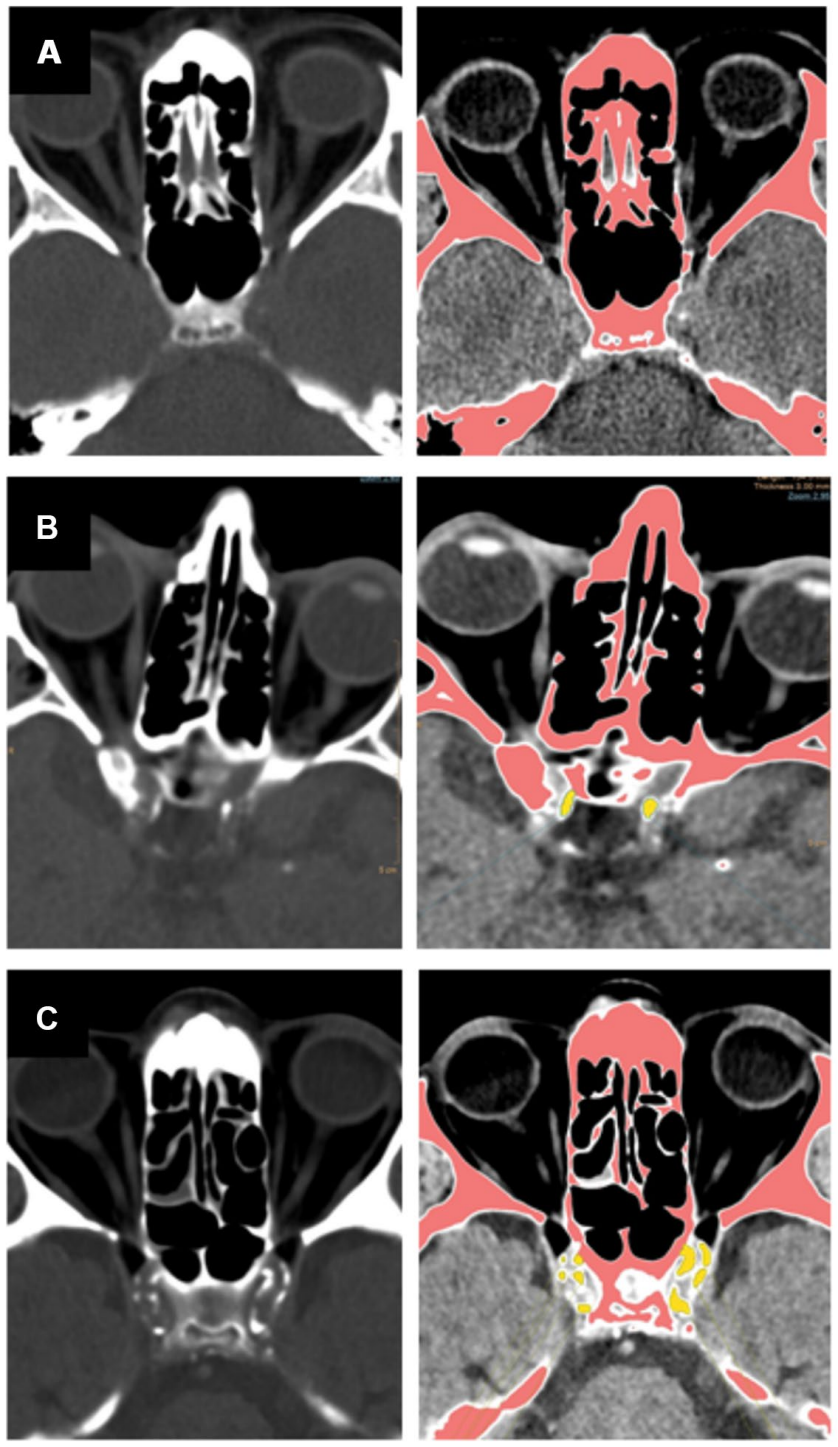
Presented are demonstrative images showcasing quantitative analysis of the intracranial calcium score, depicting axial non-contrast CT scan images, bone window, and the semi-automated quantitative ICC analysis. Hyper-attenuated regions indicating calcifications are depicted in pink, vessel calcifications are outlined manually in yellow. Panel (A) – absence of calcifications with a low ICCS, Panel (B) – moderate burden of intracranial calcifications with a moderately increased ICCS, Panel (C) – high burden of intracranial calcifications resulting in a high ICCS.
